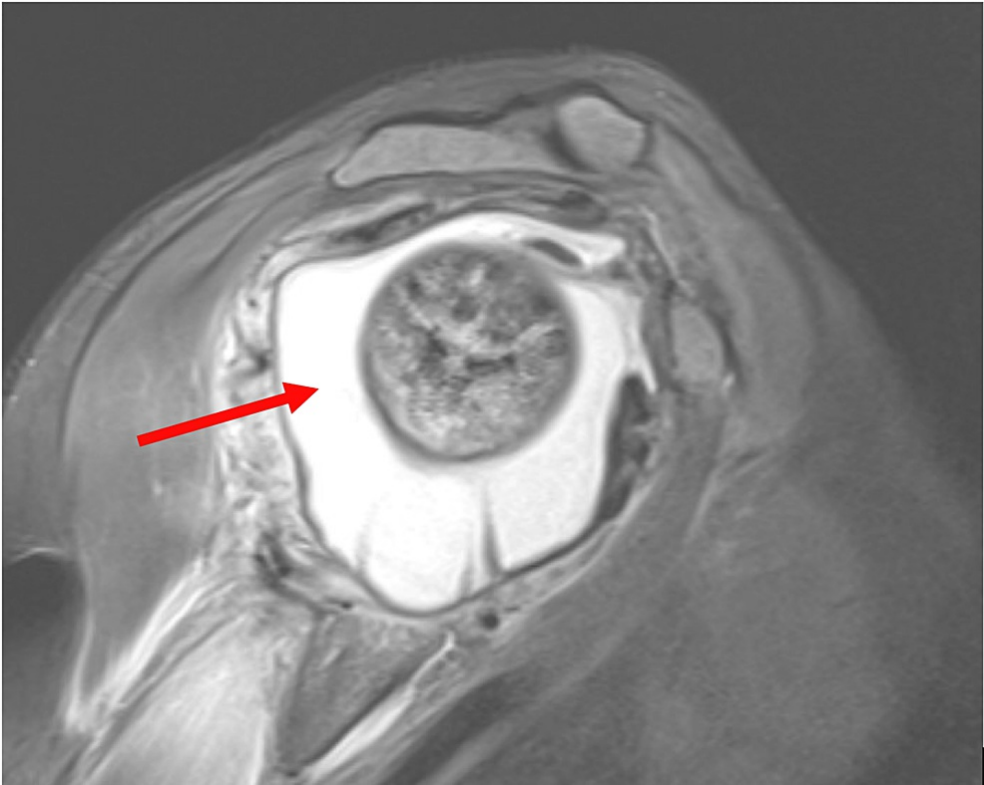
T2 MRI showing a large right glenohumeral joint effusion (red arrow) on day 10 of hospitalization, initiating joint aspiration and culture, and later antibiotic treatment.
